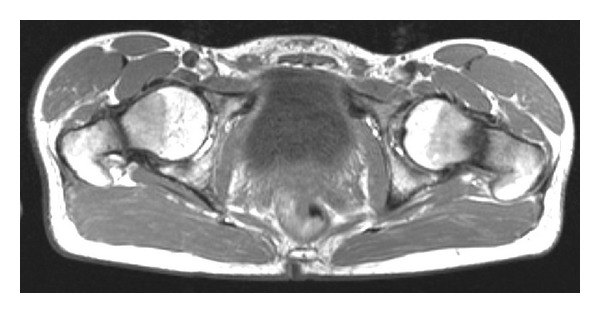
Axial T2-weighted MRI of the pelvis showing bilateral, atrophic nonunions of the femoral neck fractures.
